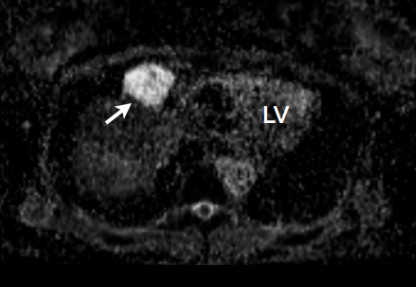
Case 1 -The ADC map using DWI CMR demonstrates a high value of the cyst contents, 3.47 × 10^-3 ^mm^2^/s. The ADC of cerebrospinal fluid measured in this patient was 3.1 × 10^-3 ^mm^2^/s.
